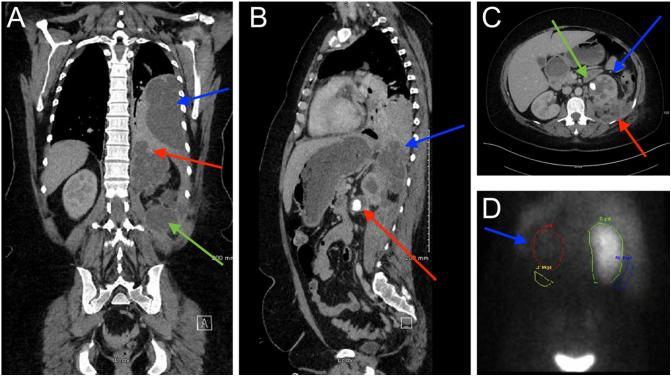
A: Coronal contrast enhanced CT demonstrating large loculated left-sided pleural effusion with extensive compressive atelectasis (blue arrow), dilated left renal calyces and perirenal collections extending superiorly through defect in the left hemidiaphragm (red arrow), and multiple pararenal organised collections (green arrow). B: Sagittal images from same CT demonstrating perirenal collections extending through the left hemidiaphragm defect to the left hemithorax (blue arrow), obstructing PUJ calculus (red arrow). C: Axial images from same CT demonstrating oedematous poorly enhancing left kidney with calyceal dilation giving multiloculated appearance (bear paw sign), consistent with XGP (blue arrow). Obstructing calculus at the left PUJ (green arrow). Multiloculated left-sided perirenal and pararenal collections (red arrow). D: DMSA image demonstrating no uptake in the left kidney (blue arrow), consistent with diagnosis of XGP. (For interpretation of the references to colour in this figure legend, the reader is referred to the web version of this article.)
